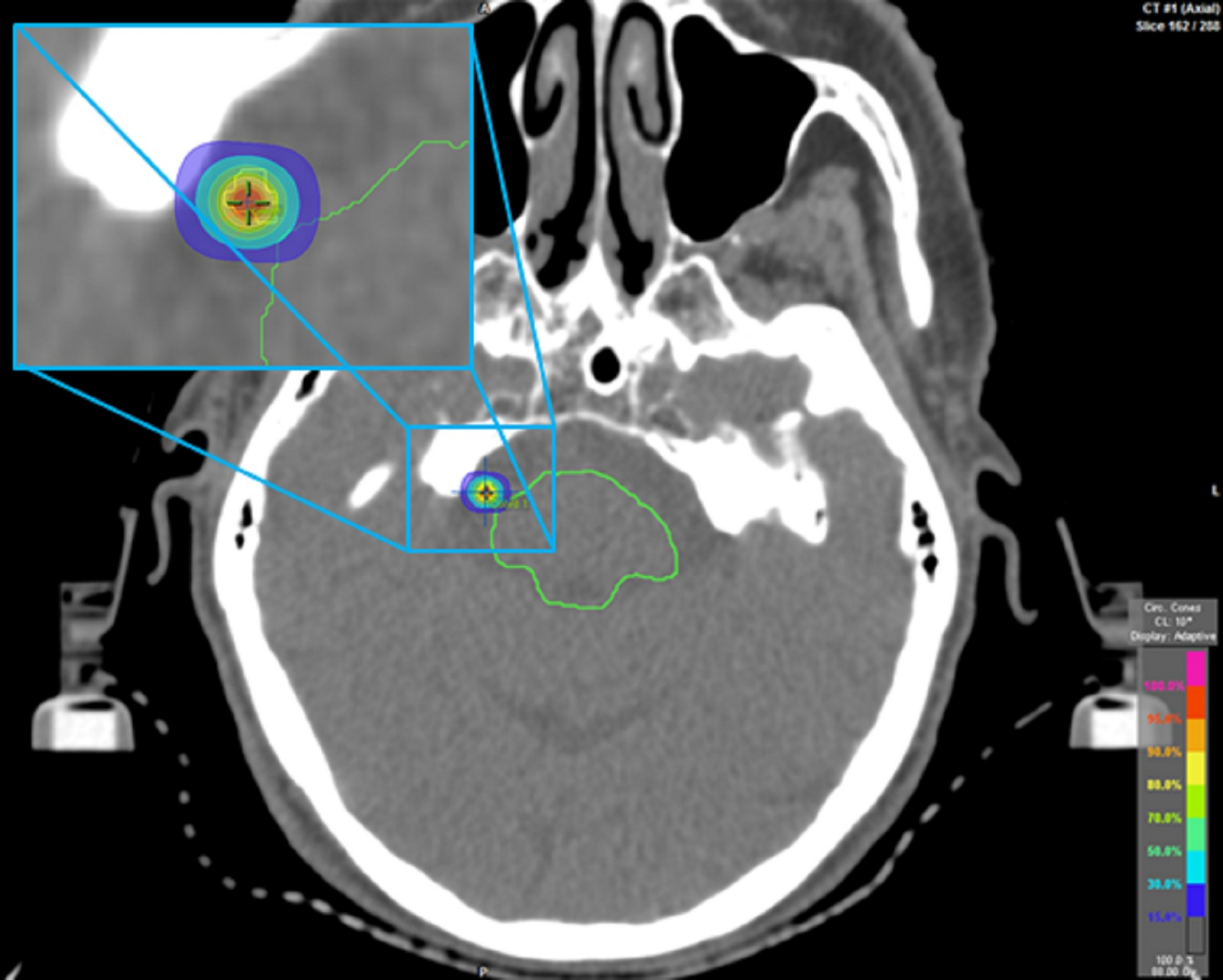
Axial Head CT. The image demonstrates the SRS treatment isocenter with 30% IDL abutting the brainstem (contoured green).
